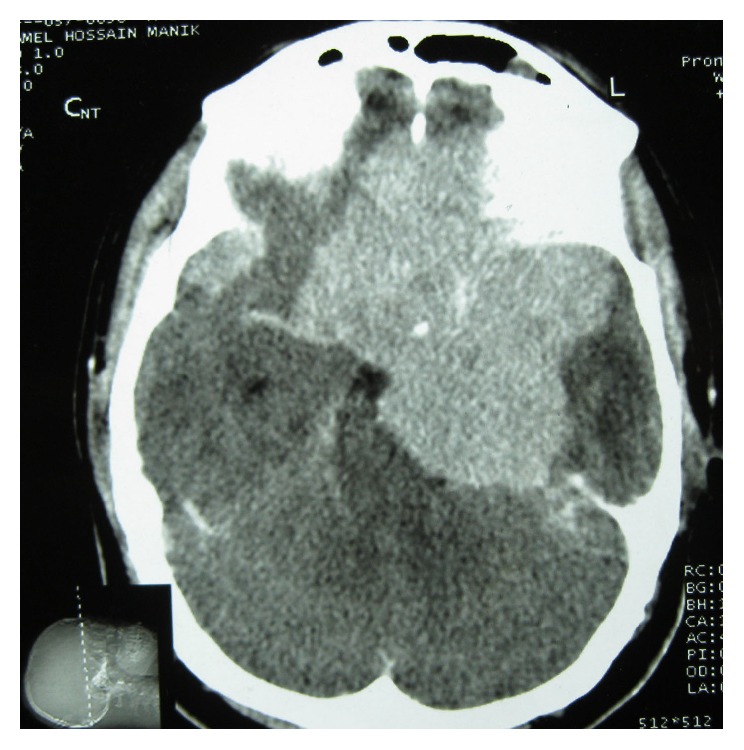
CT scan of brain showing that the lesion has encircled both optic nerves.
